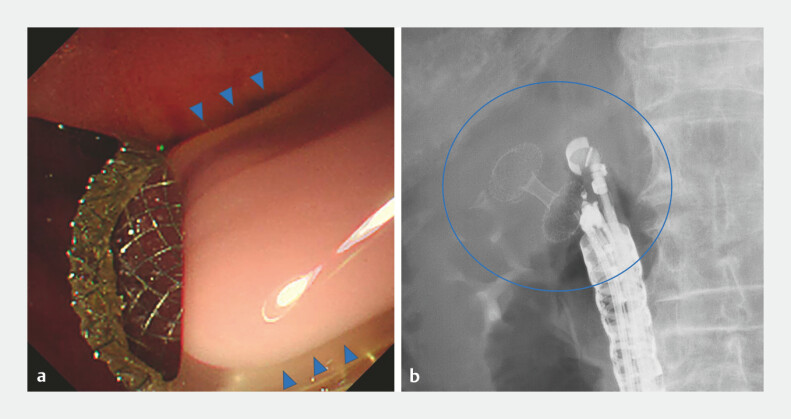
Endoscopic ultrasound-guided gallbladder drainage (EUS-GBD) for acute cholecystitis.
